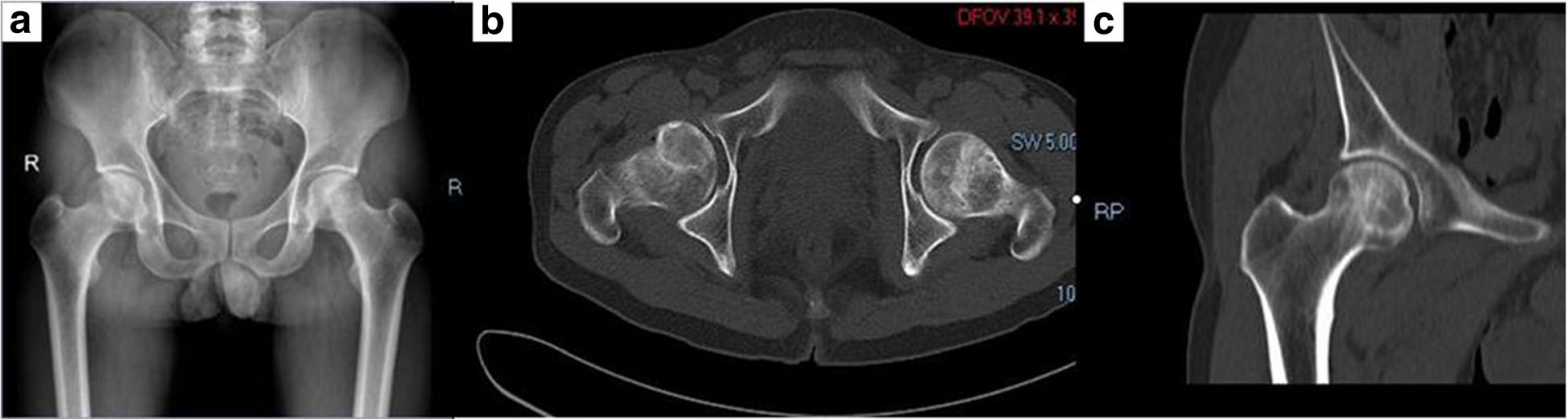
a–c Pre-operative X-ray and CT images of hip joint
